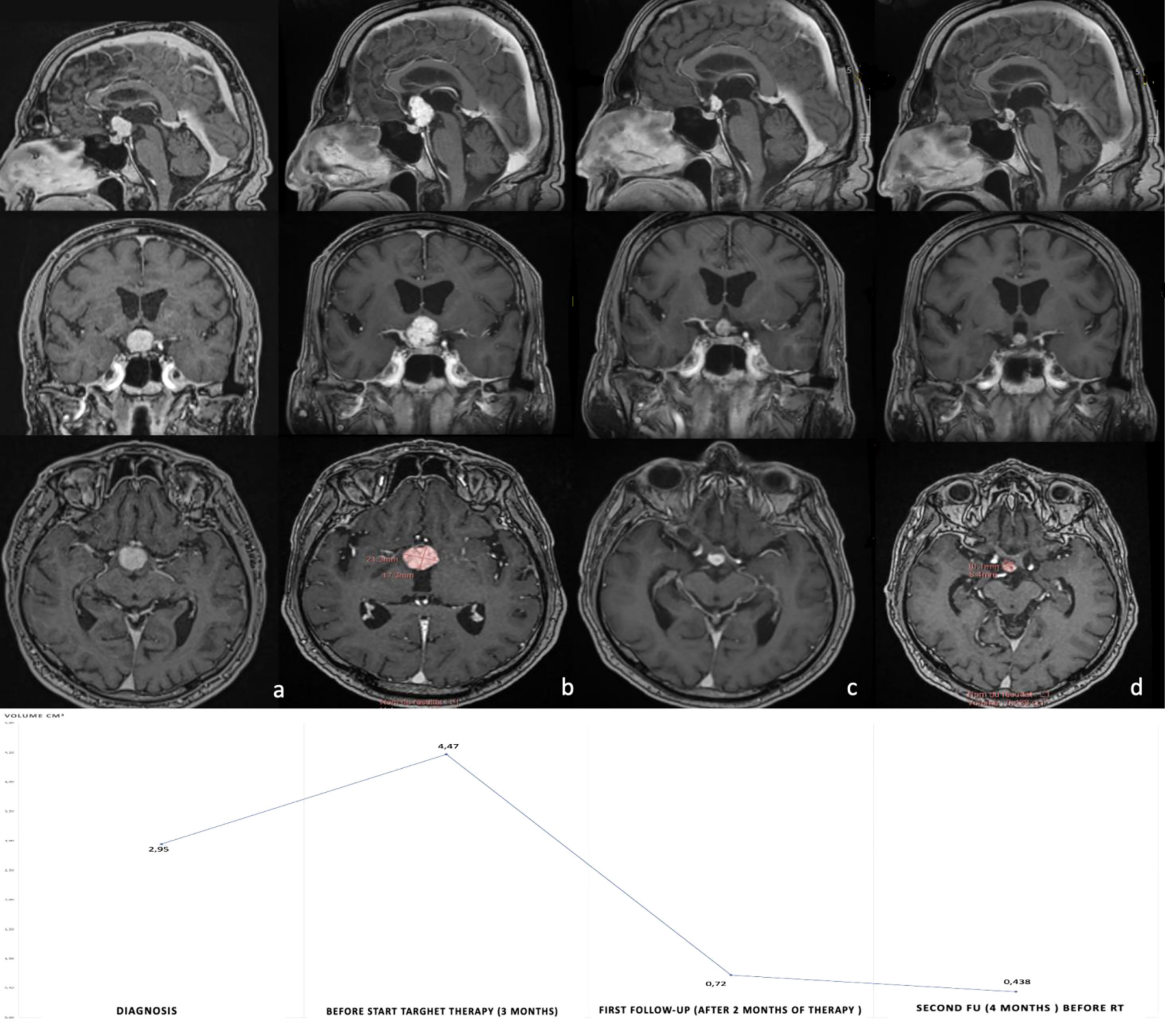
Post-gadolinium axial, coronal and sagittal T1WI MRI images of case 2. (A) Postcontrast T1-weighted image shows large homogeneously enhanced intraventricular mass measuring 19 x 18.5 mm maximal axis and 2.945 cm^3^ volume. (B) Shows progression of the intraventricular tumor portion after trans-ventricular endoscopic biopsy (18% of tumor volume). Panels c and d show a dramatic reduction in volume at 2 months (C) and 4 months (D) after commencing combined BRAF/MEK inhibitor treatment. Note complete resolution of the mass effect on suprasellar neurovascular structures and on Monro’s foramen. Volume curve has been reported in the inferior part of figure. RT, radiotherapy.
